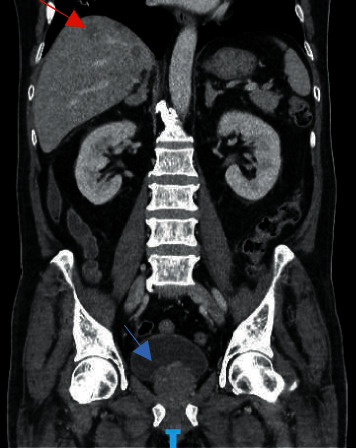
CT thorax abdomen and pelvis showing prostate cancer (blue arrow) with liver metastases (red arrow).
